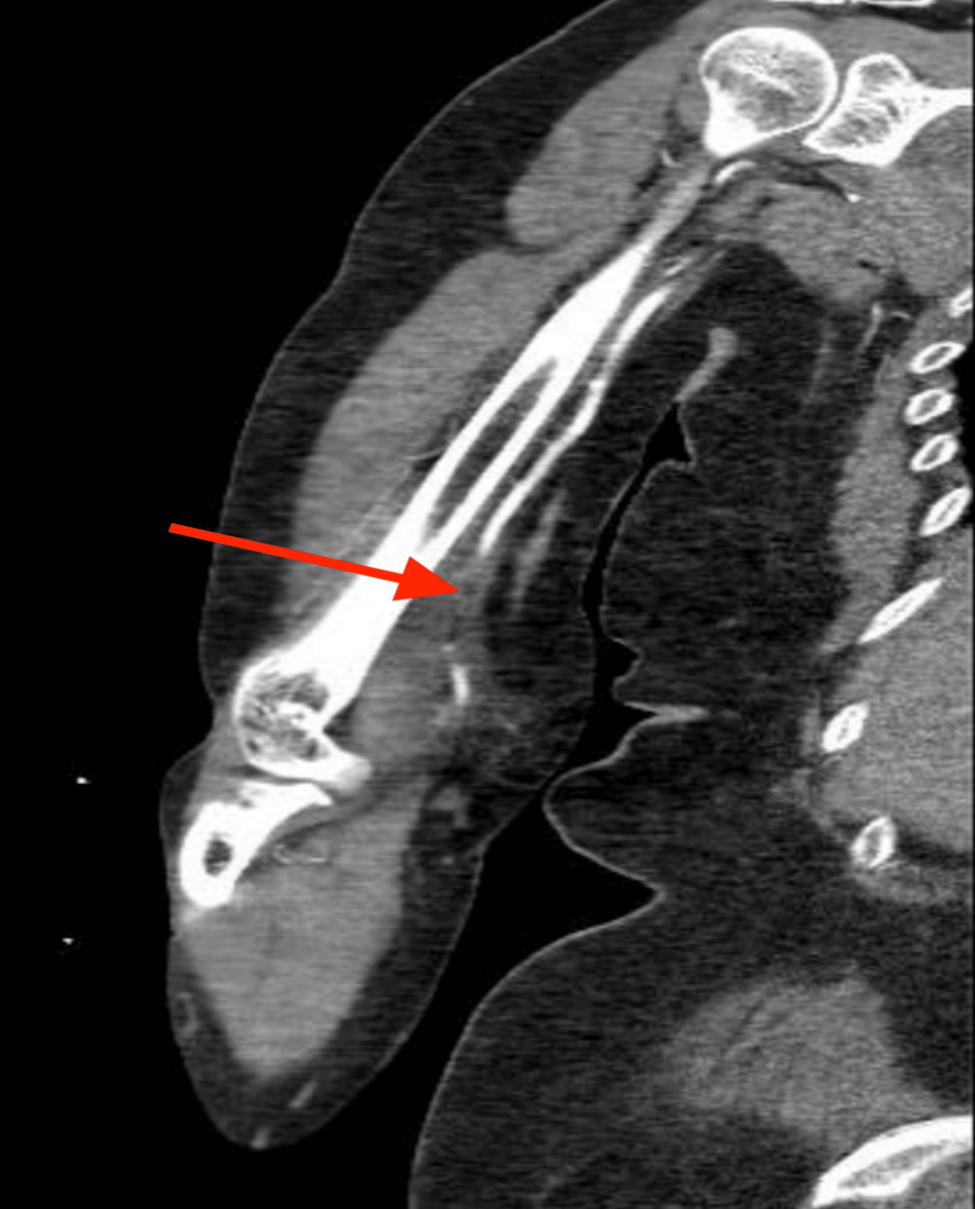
CT angiogram CT angiogram of the right upper extremity demonstrated an abrupt contrast cutoff within the brachial artery, consistent with acute arterial occlusion. There was no visualization of distal reconstitution, indicating impaired perfusion beyond the site of occlusion.
